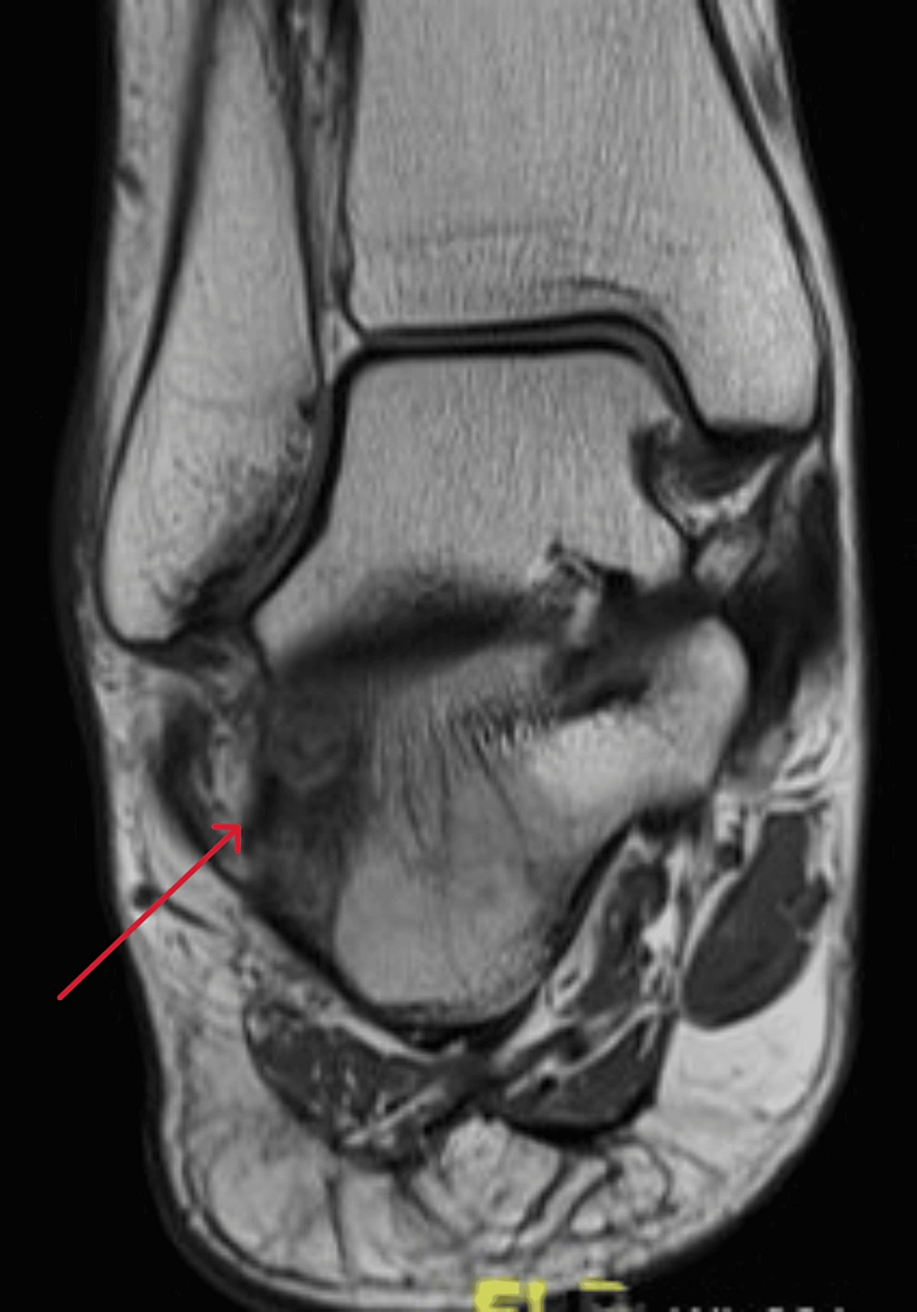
Coronal view MRI T1 window of the right ankle at the time of presentation MRI: magnetic resonance imaging
